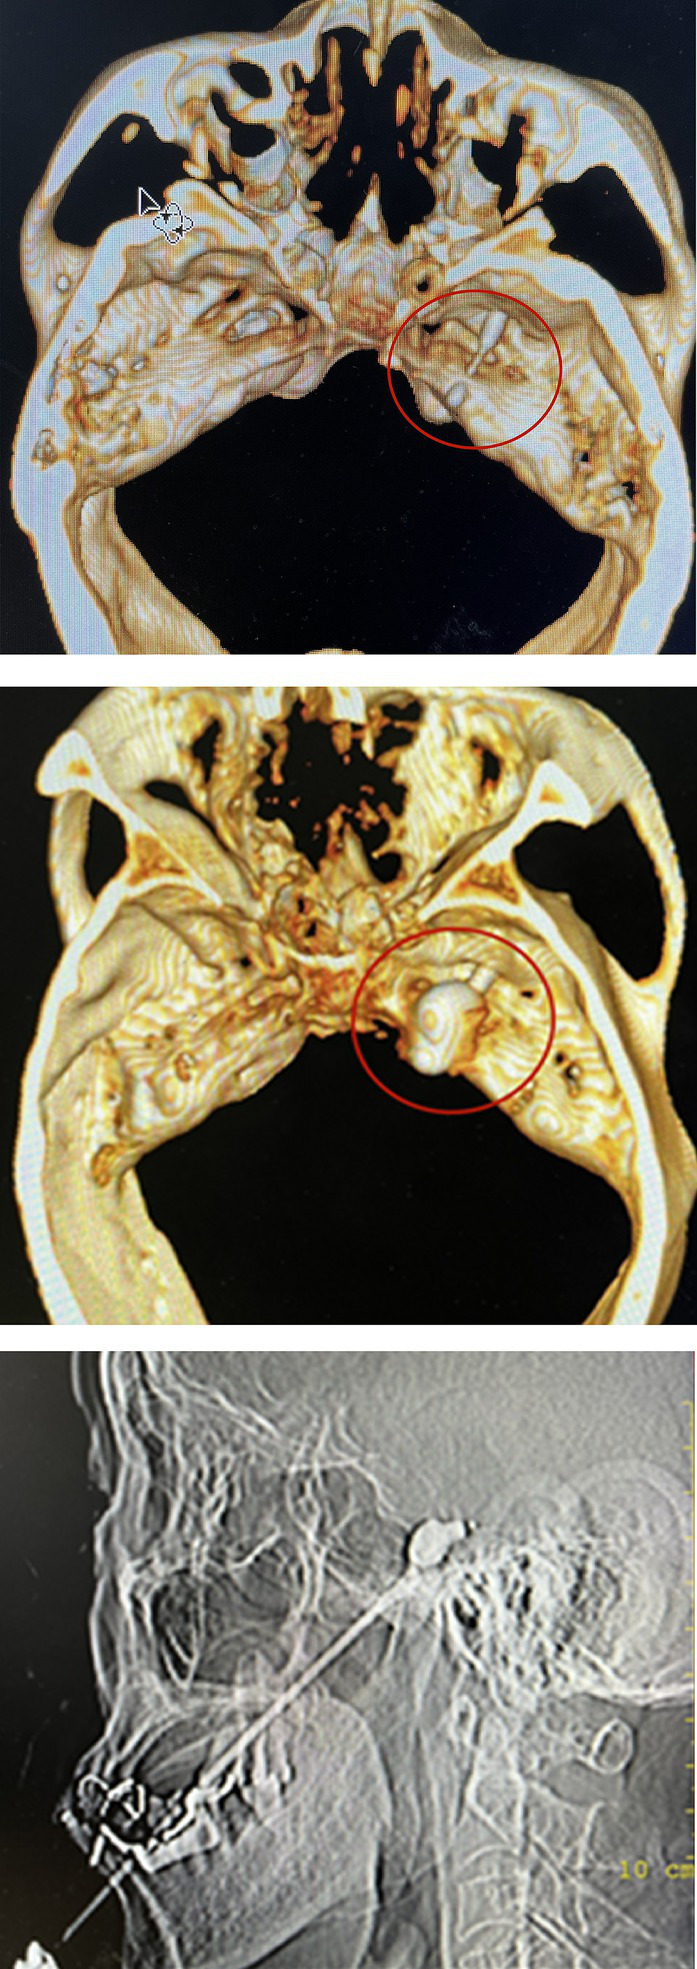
The location of balloon catheter can be determined by inserting balloon catheter under CT-guided. After the iohexol was injected, a “pear shaped image” was displayed on lateral CT imaging, indicating successful surgery.
